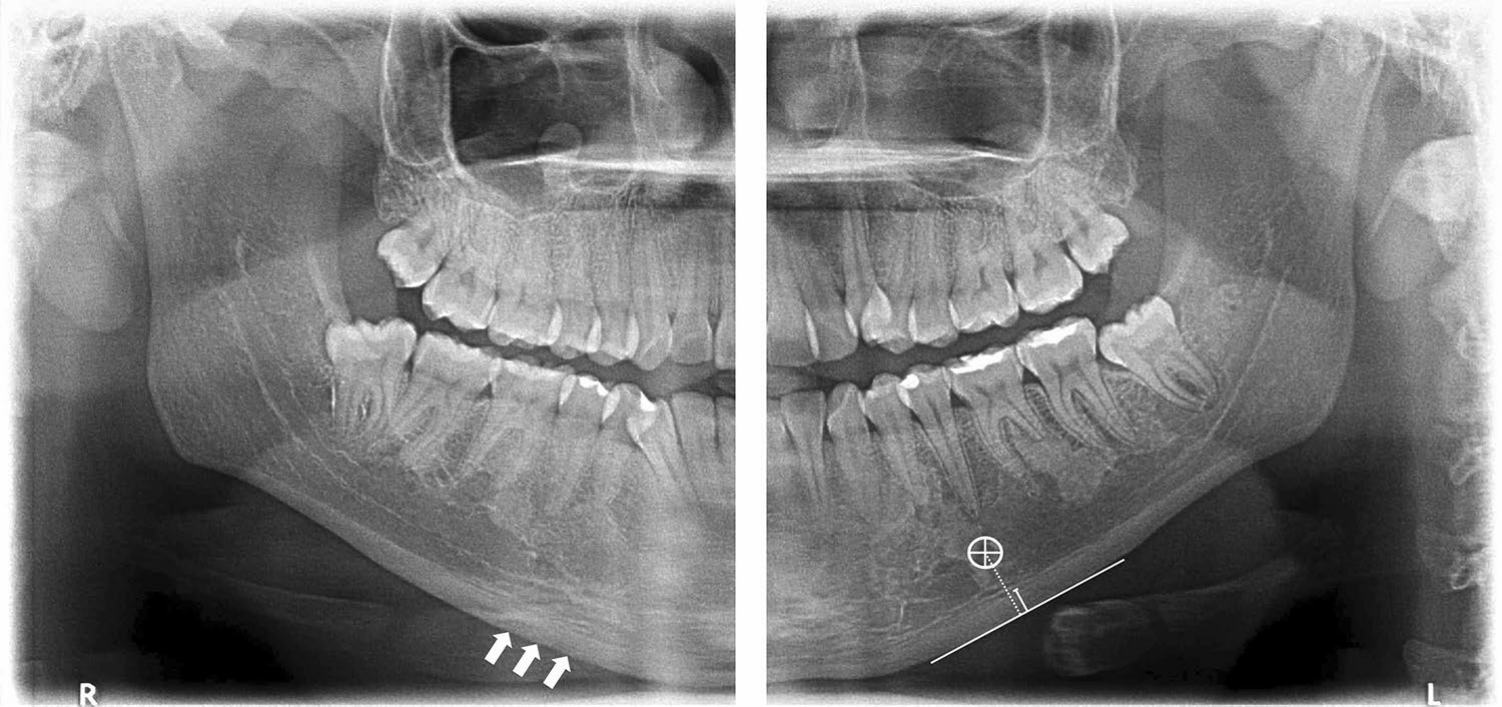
Panoramic radiograph representing the MCI assessment and tracing for MCW measurement. The left cropped DPR shows the inferior cortical border of the mandible that was evaluated qualitatively as C1 = considered homogeneous, C2 = mild erosions and C3 = multiple erosions appearance, clearly porous. The right cropped DPR radiograph showing the mandibular cortical width index. The mental foramen is delimited on the radiograph, and two parallel lines are drawn to demarcate the upper and lower edges of the mandibular cortex. A third line is drawn in the center of the mental foramen and perpendicular to the two cortical lines. The cortical width is determined by measuring the parallel line that surrounds the two structures.
